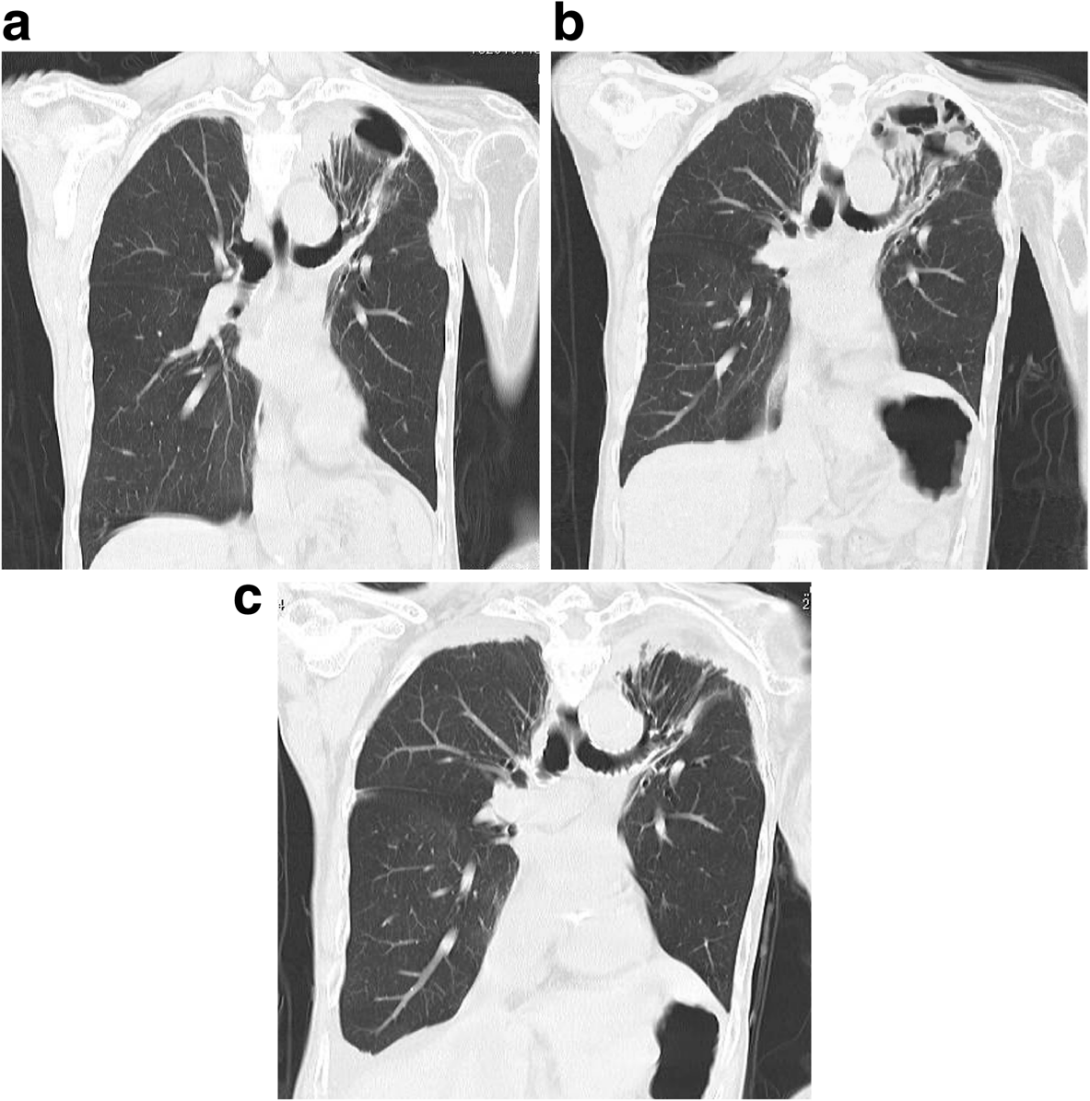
Computed tomography scans before the surgery and at 2 and 7 years after the surgery. a: The upper left lobe collapsed, and the peripheral B1/2 and B3 bronchi or bronchioles were open at the base of the fistula. b: Emphysema was visible inside the omental filling, but there was no communication between emphysema and the skin surface. c: Emphysema resolved. The grafted omentum and surrounding scar tissue were visible
